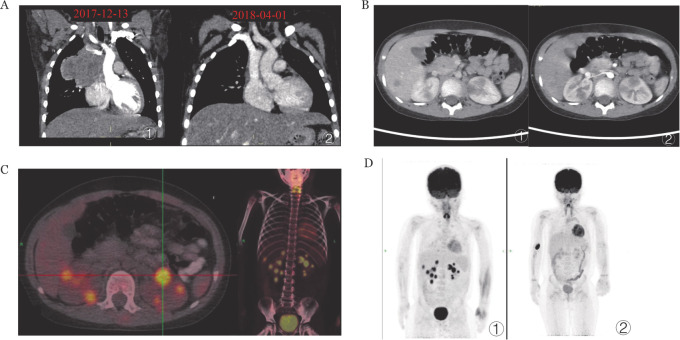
治疗前后病灶影像学变化 A ①初诊时右前上纵隔旁不规则占位病变；②化疗中期评估纵隔瘤灶较前明显退缩； B ①维持治疗1年，肝右叶新发一枚稍低密度结节灶；②左、右肾各新发数枚低回声结节灶； C、D ①PET/CT示肝及双肾多发低密度结节； D ②停化疗后复查PET/CT肝及双肾异常高代谢灶消失
